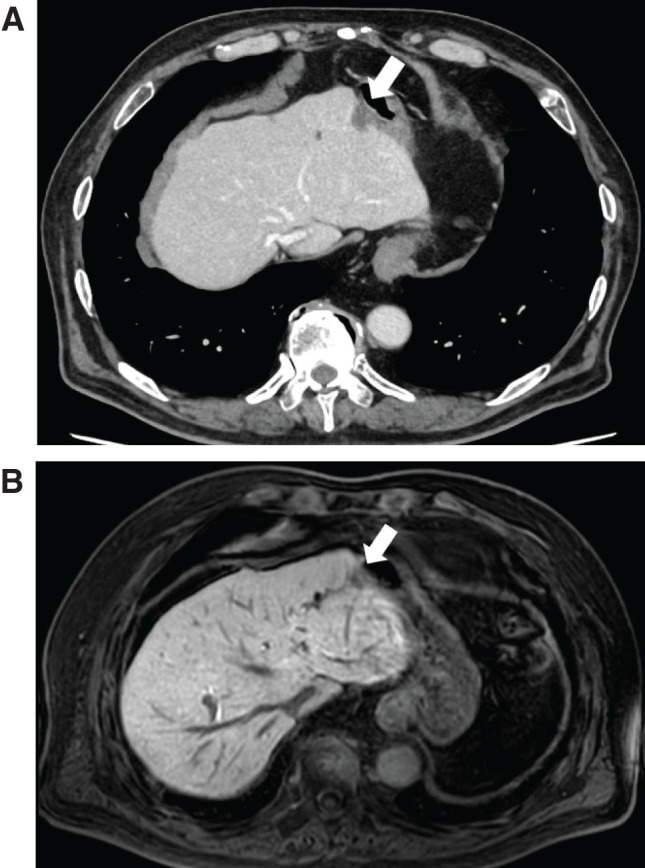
Imaging 4 months after laparoscopic cholecystectomy with gallbladder bed resection. (A) Surveillance axial CT showing a tumor in segment 3 (white arrow). No bile duct dilation was observed. (B) Axial T1-weighted MRI showing a tumor in segment 3 (white arrowhead).
